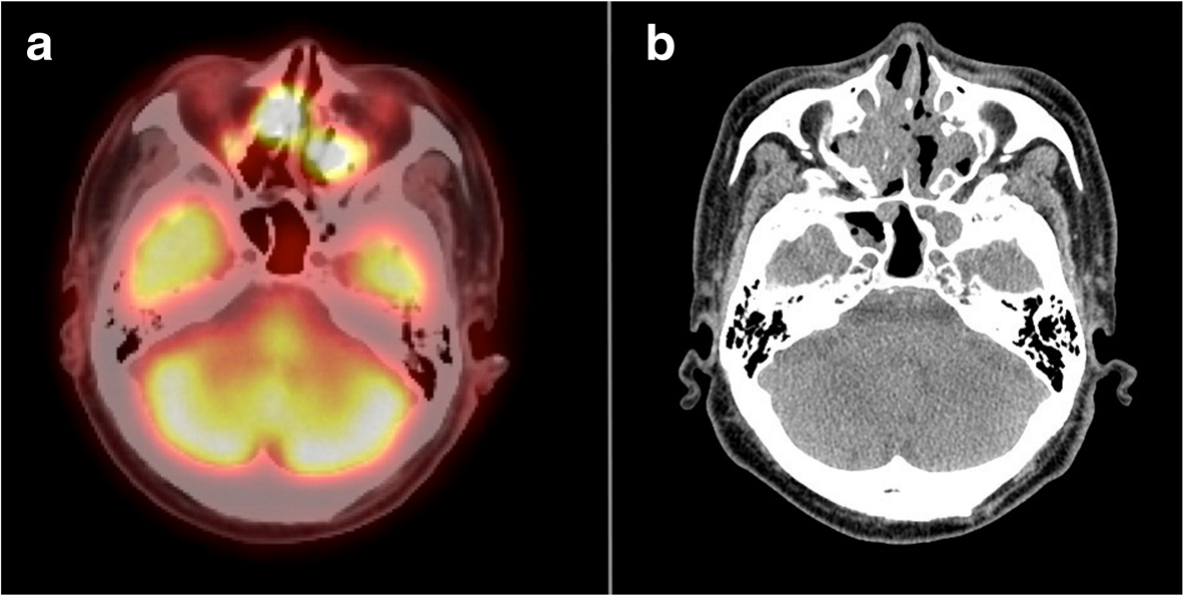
a Positron emission tomography/computed tomography showed abnormal uptake of ^18^F-Fluorodeoxyglucose in bilateral ethmoid sinuses. b Computed tomography scan showed masses in the nasal cavities and the paranasal sinuses
